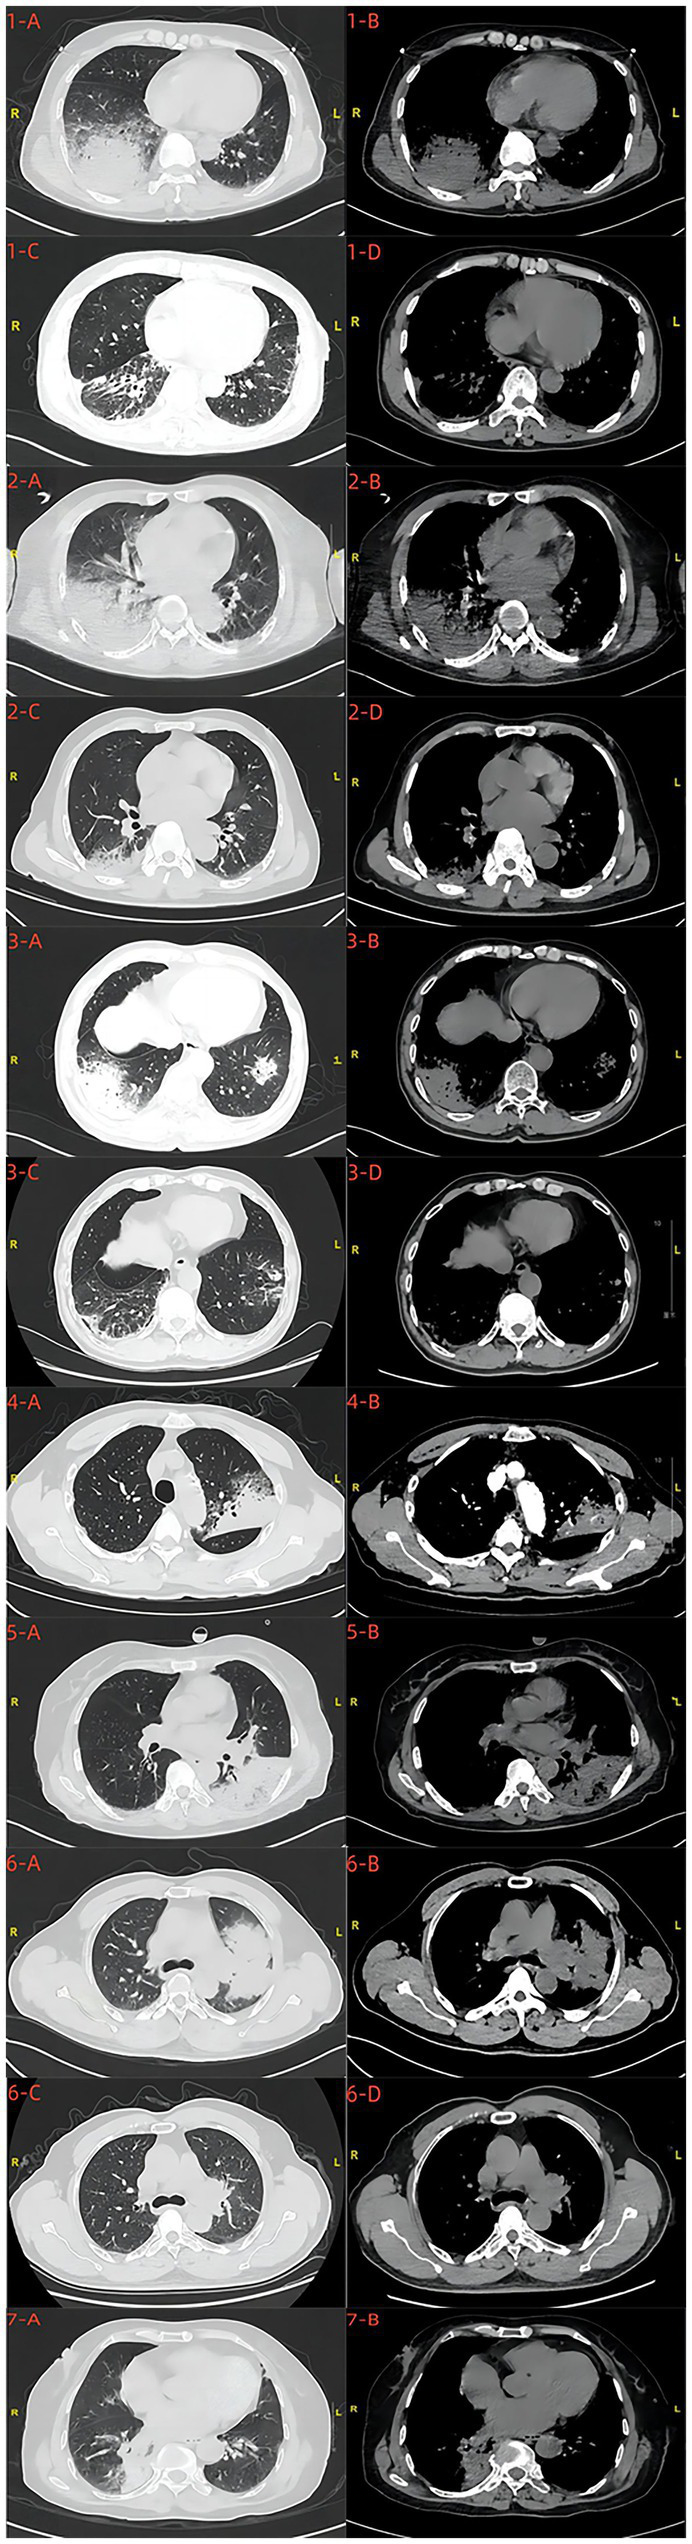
Chest CT scans before and after treatment 1-A,B,C,D; 2-A,B,C,D; 3-A,B,C,D; 6-A,B,C,D were, respectively, the chest CT scans of cases 1, 2, 3, and 6 patients before and after treatment. 4-A,B;5-A,B;7-A,B were, respectively, the chest CT scans of cases 4,5 and 7 patients before treatment.
